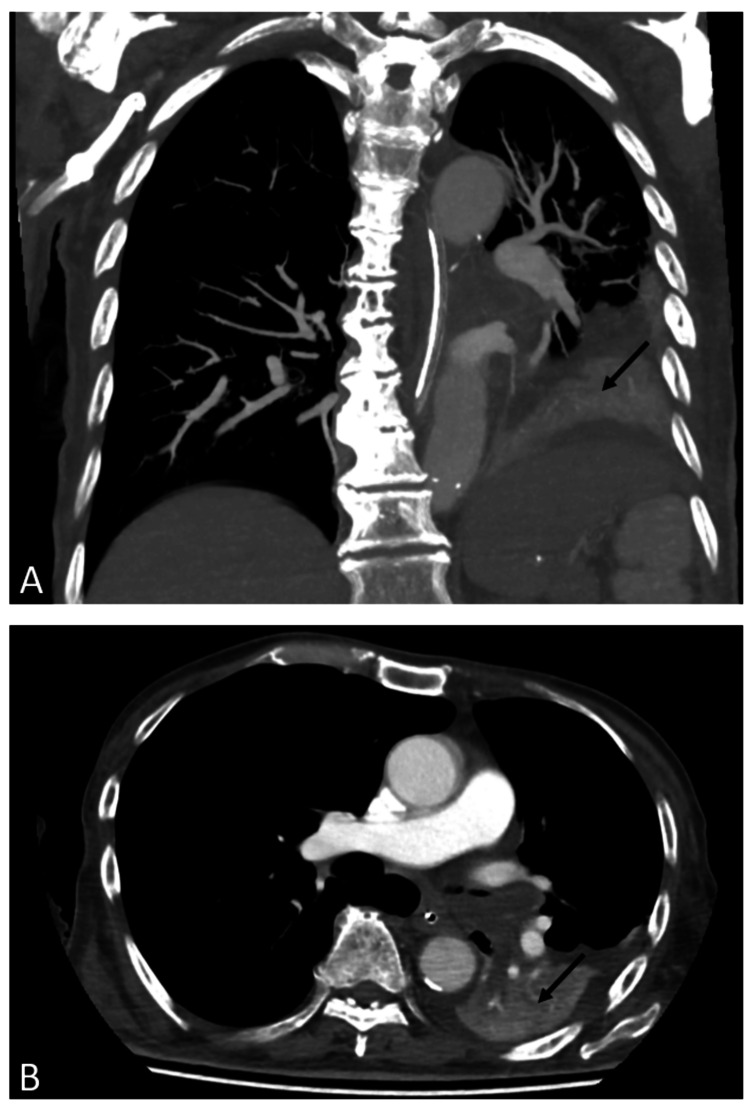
Coronal (A) and axial (B) computed tomography pulmonary angiography show an ill-defined, heterogeneous, hypodense lesion at the anteromedial segment of the left lower lobe (approximate size: 2.6 × 4.3 cm).
